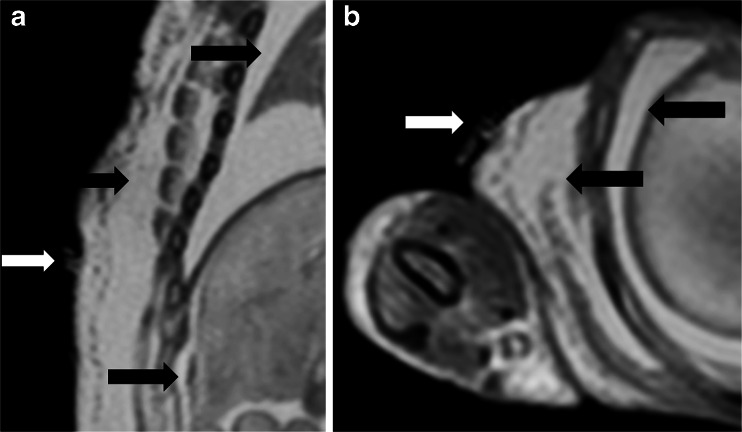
Maceration. Coronal (a) and axial (b) T2-weighted postmortem magnetic resonance imaging body imaging of a 26-week gestation fetus with skin slippage due to maceration. Skin fragments can be seen on the body surface (*white arrows*) with significant underlying subcutaneous oedema around the upper limb and thorax, a large pleural effusion and ascites (*black arrows*)
